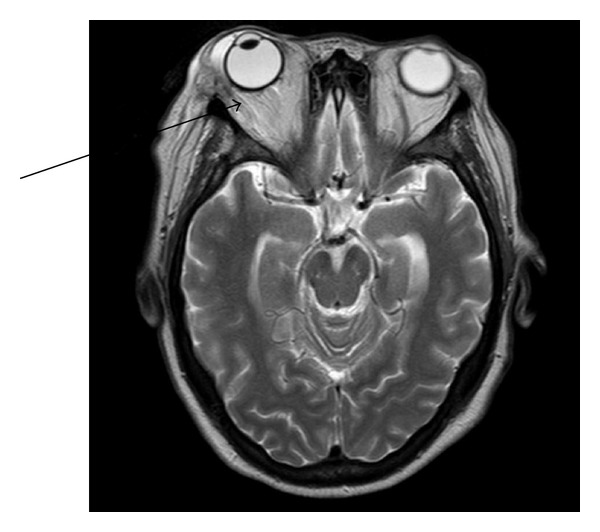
MRI of the orbit showing involvement of the greater wing of the sphenoid along with right orbital extension, involving the lateral rectus muscle and the lateral wall of the orbit as well as a right-sided preseptal soft tissue swelling and thickening.
